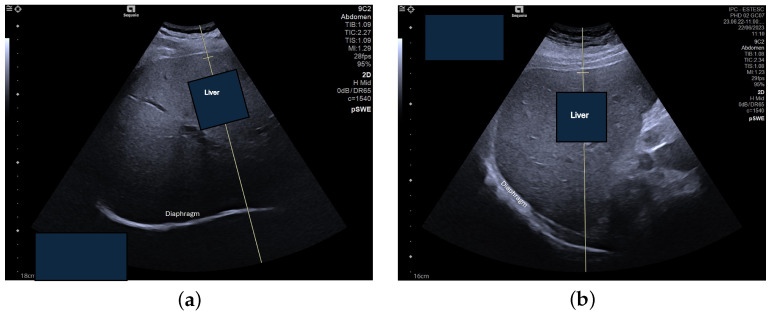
Mode B intercostal scan of the right lobe of the liver, acquired with the Acuson Sequoia ultrasound system, showing the liver: (a) without steatosis, (b) with steatosis.
